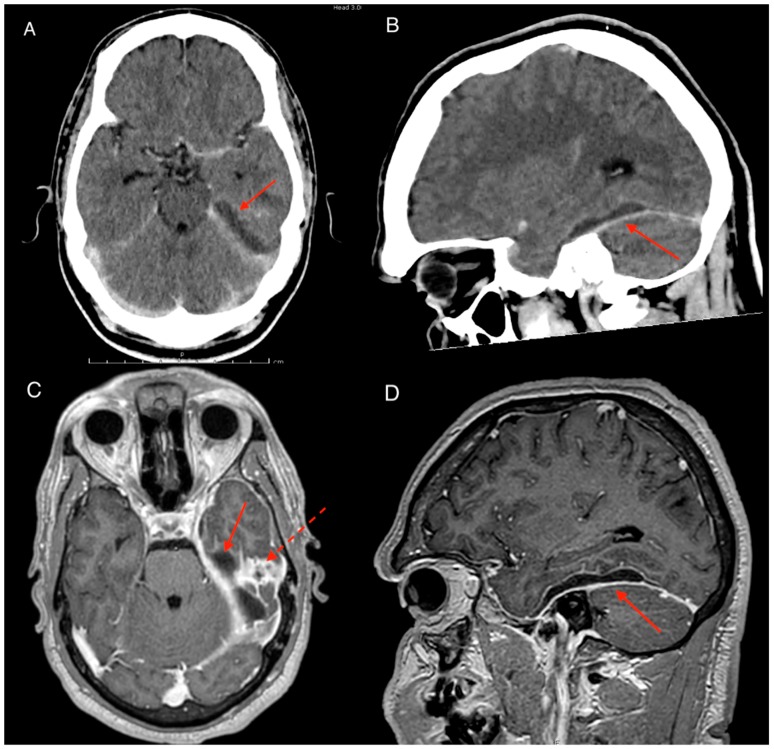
Imaging on presentation, contrast computerized topography (CT) and magnetic resonance imaging (MRI) brain T1 contrast axial and sagittal views. (A) Axial CT scan with contrast showing subdural collection in the left middle cranial fossa (red arrow). (B & D) Sagittal CT with contrast and MRI T1 with contrast showing collection above the tentorium. Note minimal contrast enhancement of the collection with no clear evidence of cerebral oedema representing early stages of intracranial subdural empyema (ISE). (C) Axial MRI T1 with contrast. Note area of contrast enhancement at the floor of middle cranial fossa on the petrous part of temporal bone (dotted arrow) correlated with the dural defect found intraoperatively.
